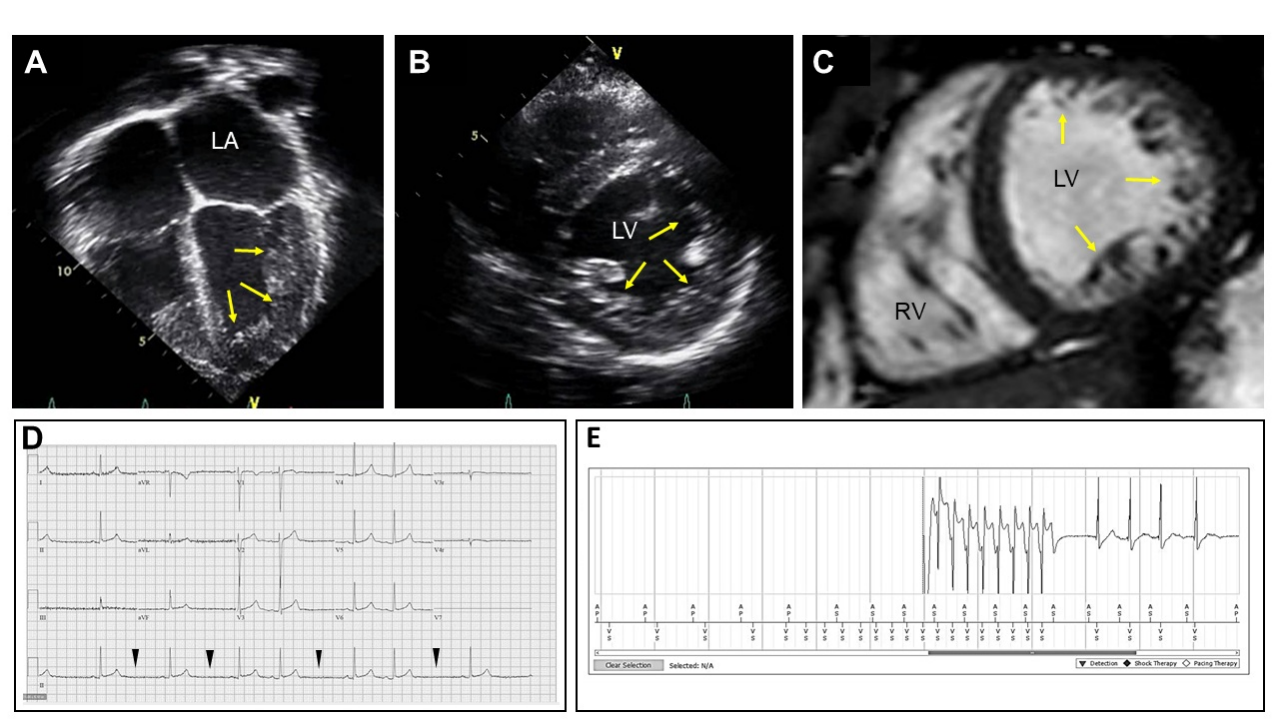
Results of echocardiographic, cardiac magnetic resonance (CMR)
